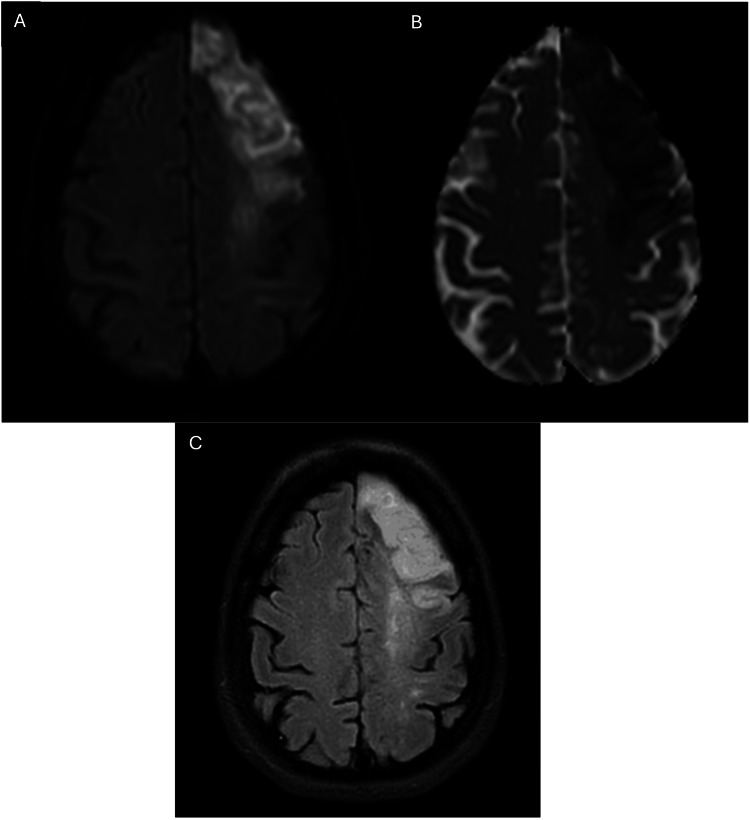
MRI of the brain showing: (A) Axial DWI image (b=1000), with corresponding low signal on the axial ADC image (B) demonstrating a left anterior cerebral artery (ACA) territory infarct. (C) The corresponding axial FLAIR image shows an increased signal at the same region. DWI: Diffusion-weighted imaging; FLAIR: Fluid-attenuated inversion recovery; ADC: Apparent diffusion coefficient
